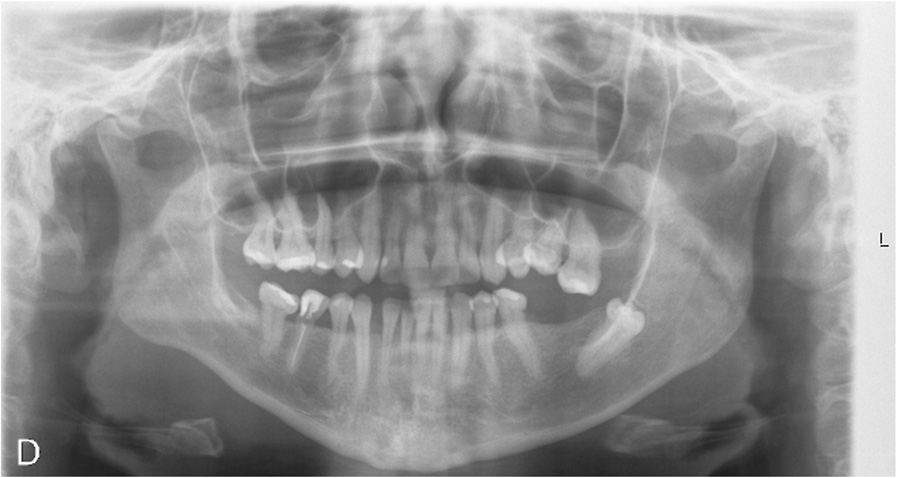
Panoramic radiography of the sister, non-affected.
